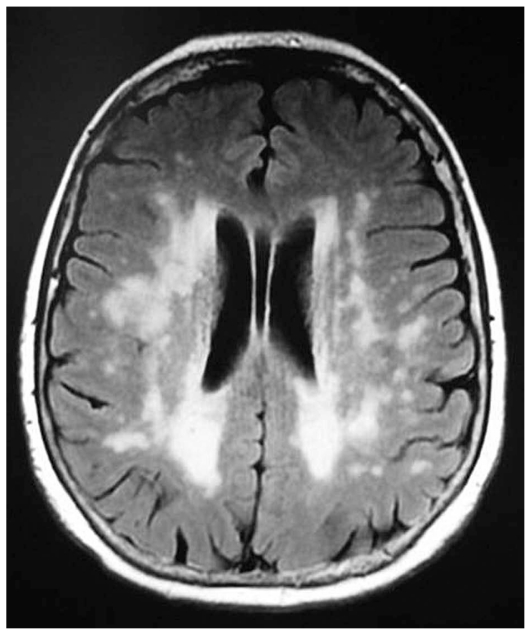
Neuro-sarcoidosis: multiple granulomas in the white matter of both brain hemispheres (contrast enhanced T1-weighted MRI image). Focal parenchymal lesions are T2-hyperintense and T1-isointense (with homogeneous contrast enhancement, as in this figure).
